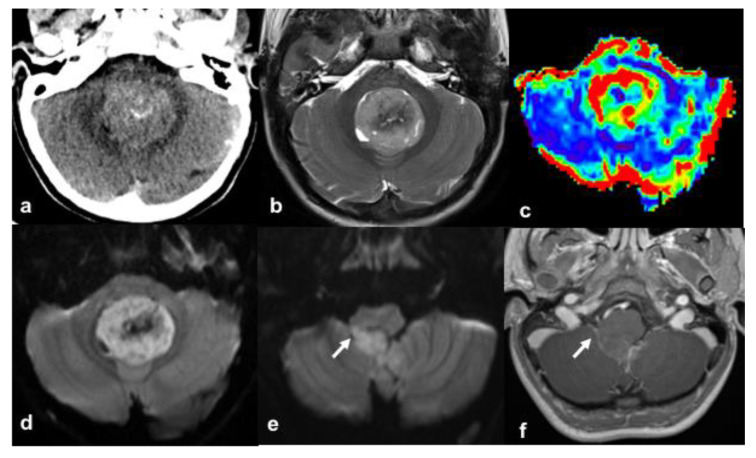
Axial computed tomography (CT) (a) and magnetic resonance imaging (MRI) (T2w (b); arterial spin labeling (c); diffusion weighted imaging (d,e); GdT1 (f)) axial images. CT study shows a fourth ventricle mass with lesional mineralizations (a). The tumor has a heterogeneous signal on MRI (b), increased perfusion (c), restriction of the diffusivity (d), and mild contrast enhancement (f). Furthermore, the involvement of the right foramen of Luschka is noted (arrow, (e,f)).
